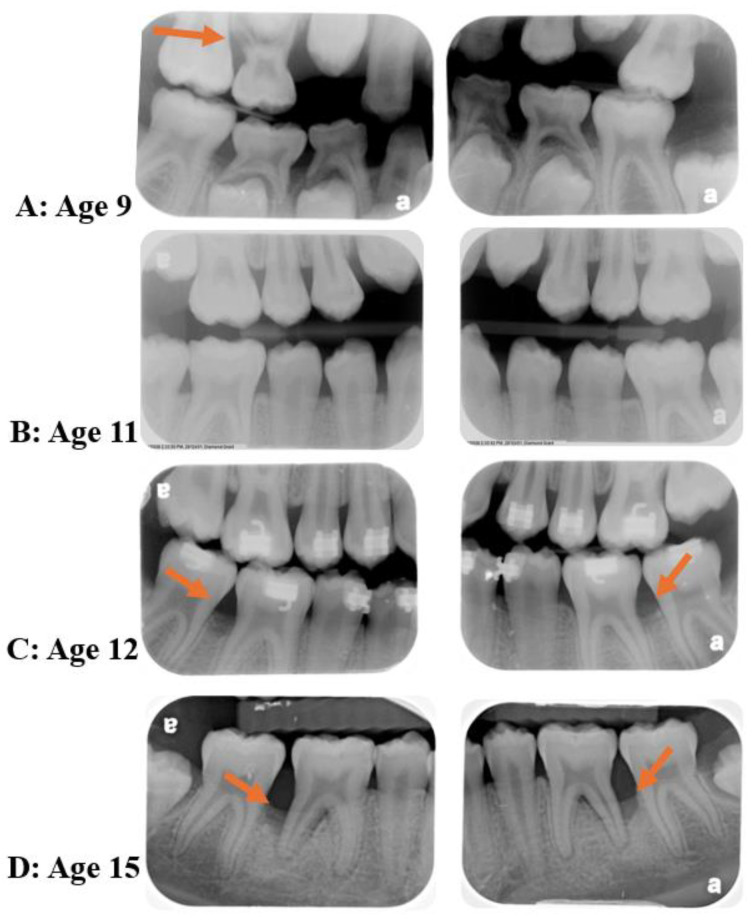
Clinical Case: The patient was referred to periodontal treatment at age 15 with significant vertical bone loss on #19 and 30. Retrospective radiographic analyses of the permanent and primary dentitions show evidence of bone loss in the upper (orange arrow) and lower primary molars at age 9 (A), healthy permanent dentition at age 11 (B), and beginning of bone defects in permanent dentition at age 12 and 15 (C,D); #19 and 30 distal surfaces) (Source of the image [10]).
